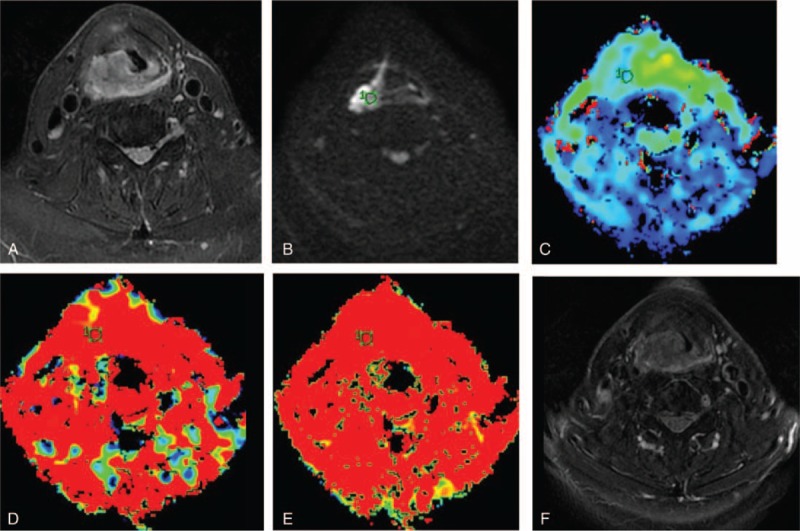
A 58-year-old man with pyriform sinus and posterior pharyngeal wall carcinoma, nonresponse to induction chemotherapy. (A) Transverse T_2_-weighted imaging. (B) Transverse diffusion weighted imaging (DWI) map obtained with *b* = 800 s/mm^2^. (C–E) The mean pretreatment *D*^∗^, *D*, and *f* values were calculated as 45.34 × 10^–3^ mm^2^/s, 0.872 ×10^–3^ mm^2^/s and 51.21% in nonresponder, respectively. (F) Transverse T_2_-weighted imaging show that tumor mass was slightly reduced (<30% reduction) after 2 cycle induction chemotherapy. DWI =  diffusion weighted imaging.
